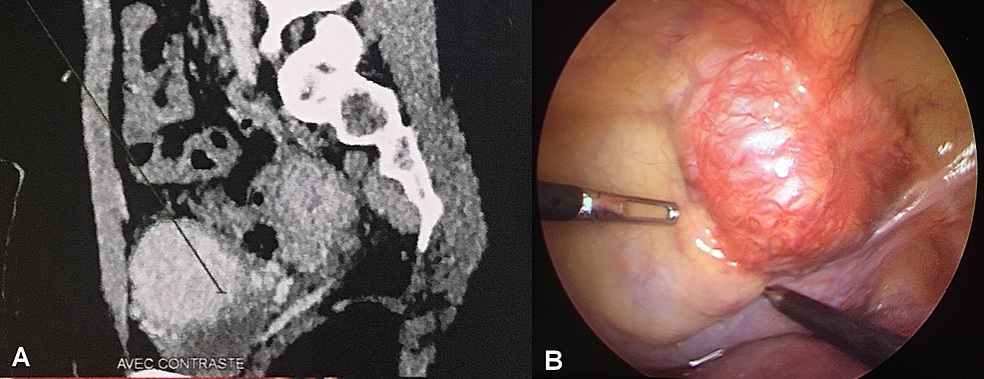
Radiomorphological and intraoperative image showing a highly vascular tumor along the dome of the urinary bladder. (A) Sagittal contrast-enhanced CT image of the abdomen. There were no signs of local invasion and regional lymph node or distant metastasis. The upper urinary tract was normal. (B) Intraoperative laparoscopic view image.
